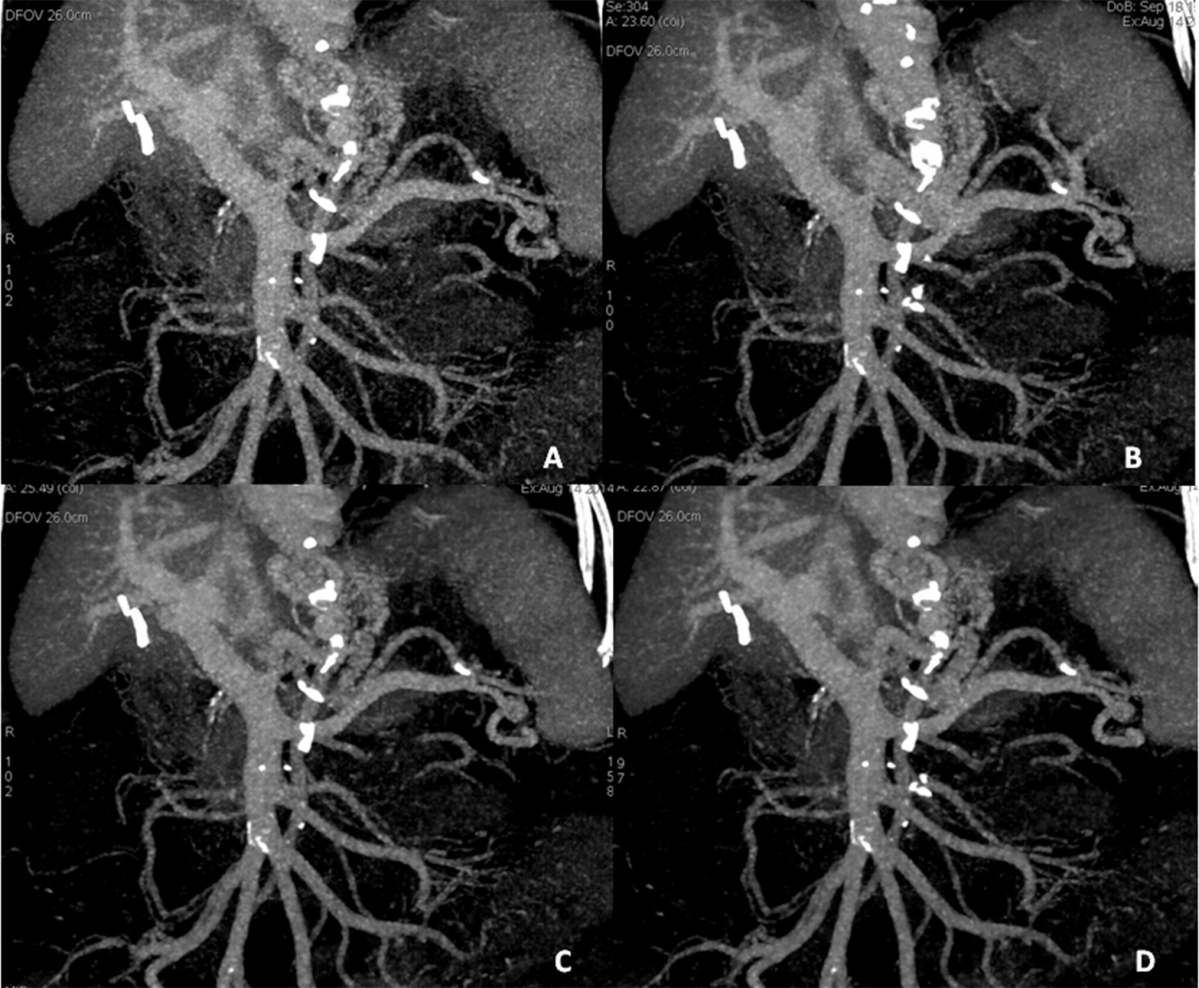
Subjective evaluation of rapid switching single source dual energy CT (ssDECT) portal venography images of a cirrhotic patient with portal hypertension secondary to liver cirrhosis with different percentage adaptive statistical iterative reconstructions (ASIRs). (A), (B), (C) and (D) were obtained with 0%, 30%, 50%, and 70% percentage ASIRs reconstruction, respectively. The same slice thickness, field of view and nearly identical angle were used for reconstruction of all images.
